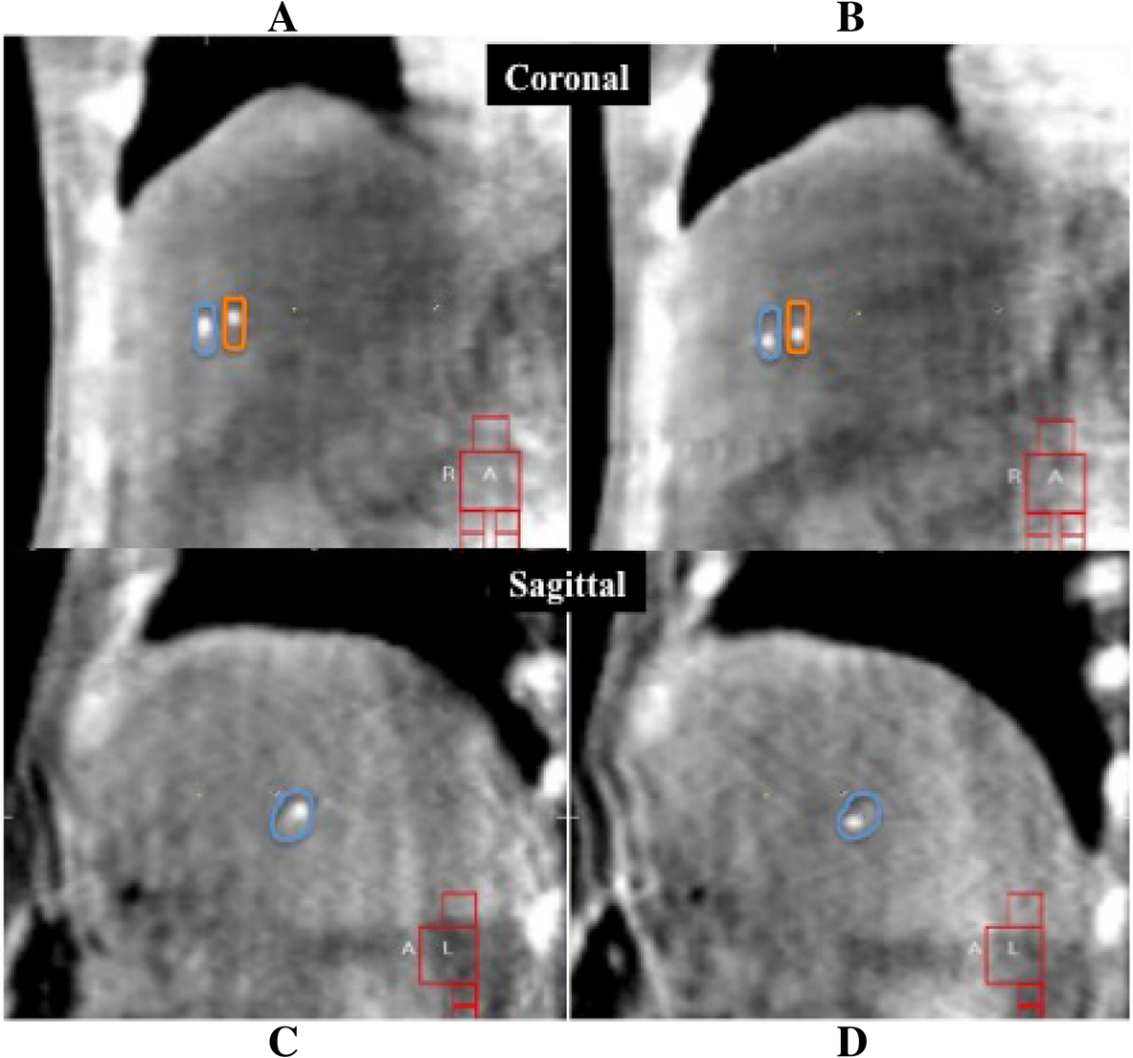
Four-dimensional cone-beam computed tomography (4D-CBCT) images of the end-exhalation a, c and end-inhalation b, d. The “internal marker target volume (IMTV)” structure (blue and orange) that reflected the motion of fiducial markers observed in 4D-CBCT were used for target localization in liver SBRT
